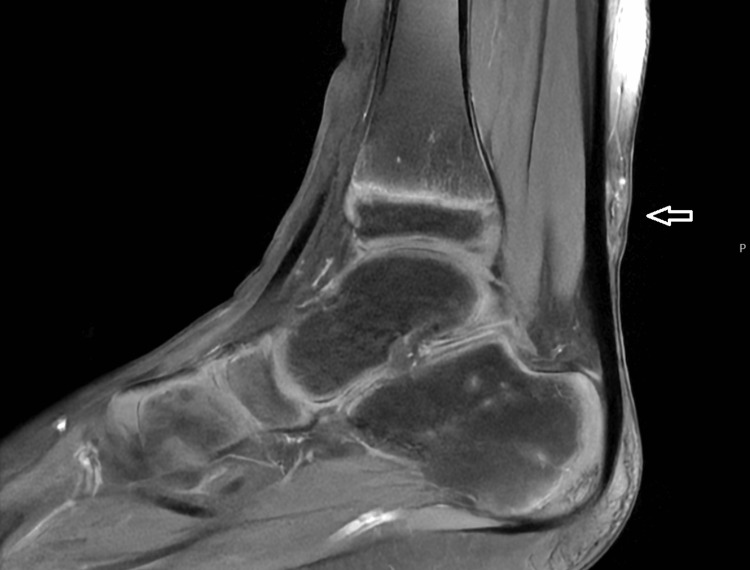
MRI scan on the sagittal plane showing a soft tissue subcutaneous mass overlying the lower third of the Achilles tendon, with areas of lower signal intensity on T1. The image demonstrates calcified tissue with mild enhancement after contrast medium administration
